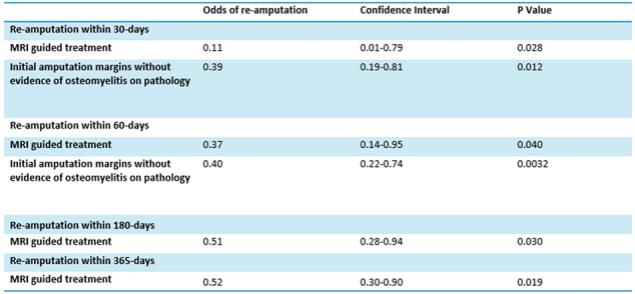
Multivariable analysis of re-amputations MRI= magnetic resonance imaging
